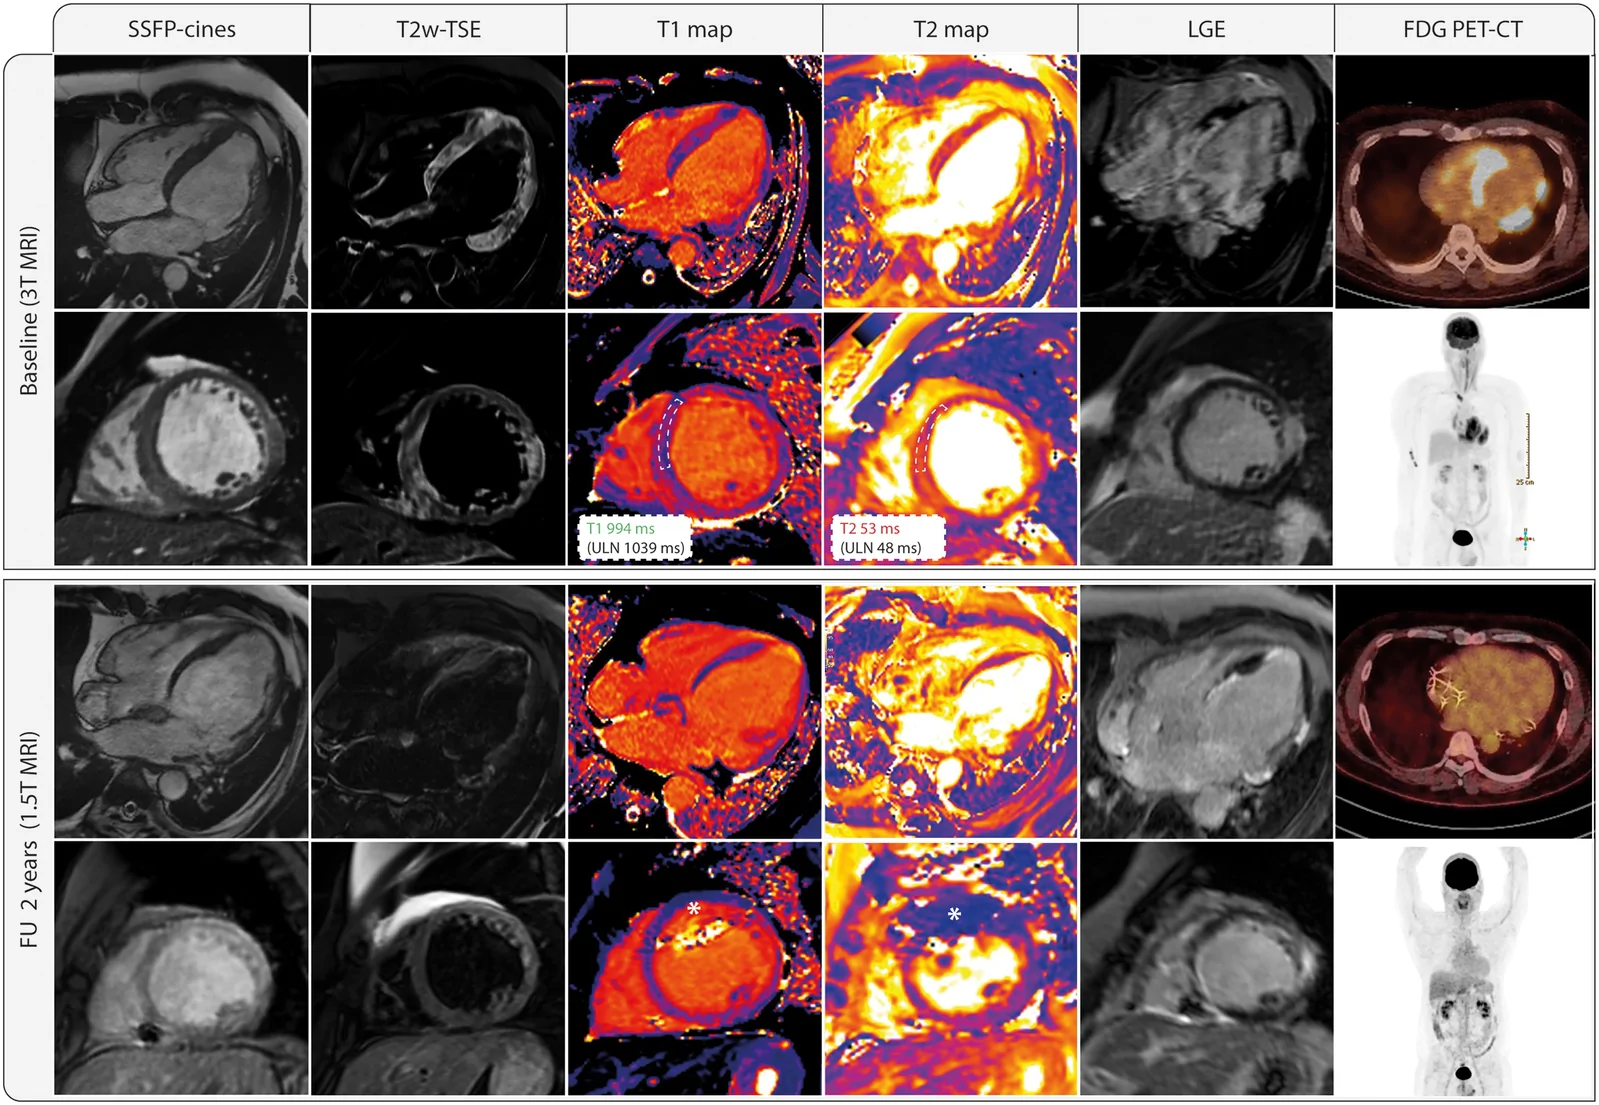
CMR findings in Case 3, a patient with cardiac sarcoidosis. Baseline CMR and fluorodeoxyglucose positron emission tomography computed tomography (FDG PET-CT) imaging: SFFP cine images showing mildly dilated left ventricle (LV) with wall thinning of the basal septum, multifocally increased signal intensity on T2w-TSE, regionally increased myocardial T1 values anterior/inferior (anteroseptal normal T1 values 994 ms) with diffusely increased T2 values (mean 54 ms, anteroseptal 53 ms), and extensive multifocal nearly transmural LGE matching the areas with increased T1 values. Lastly, FDG PET-CT demonstrating increased tracer uptake in the myocardium consistent with sarcoidosis. Serial imaging with CMR (after 11 months) and PET-CT (after 5 months) showed progressive LV dilatation on SFFP cine images; resolution of inflammation as demonstrated by normalization of T2w-TSE images and normalization of myocardial T1 and T2 values, apart from the artefact caused by the CRT-D (marked with an asterisk); and persistent multifocal pattern of LGE in the same regions as previously. Lastly, FDG PET-CT showed reduced disease activity demonstrated by decreased myocardial FDG uptake. Abbreviations as in *Figure 1*.
